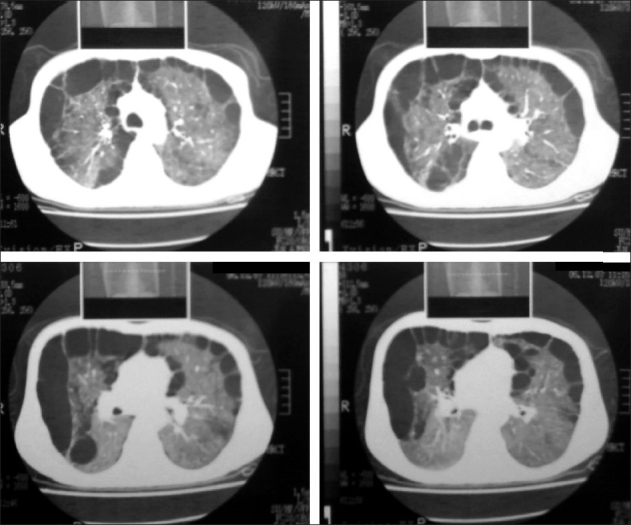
HRCT chest showing emphysematous lungs with multiple, medium- to large-sized bullous lesions
